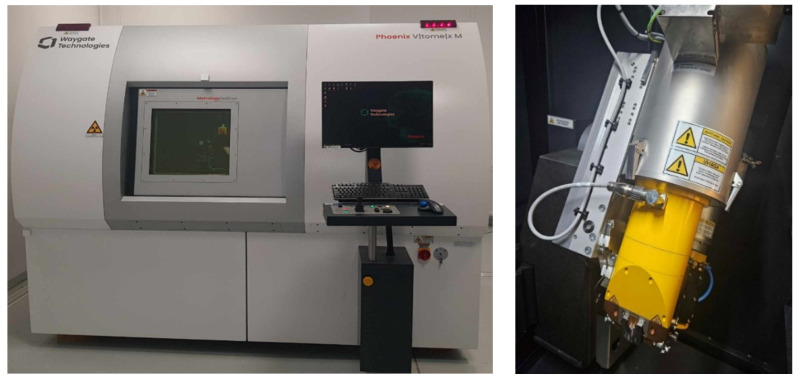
CT scanner and microtube.
